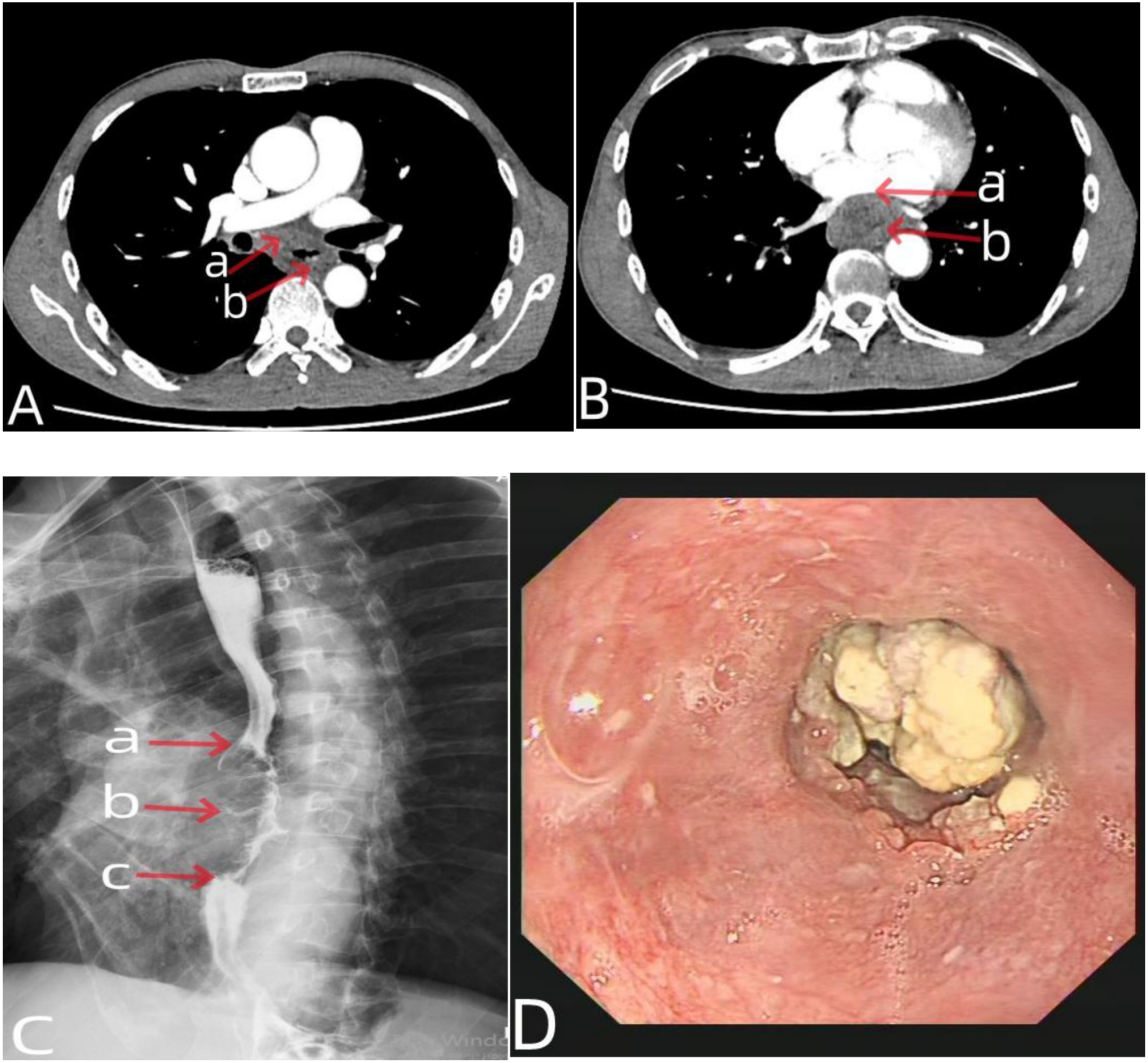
Chest CT, esophagography, and gastroscopy data of the patient before neoadjuvant chemotherapy. In (A), the arrow a represented enlarged subcarinal lymph nodes, the arrow b represented esophageal tumors. In (B), the arrow a represented the left atrium is compressed by tumor, the arrow b represented there was no clear boundary between the tumor and the thoracic aorta. In (C), the arrow a represented the upper edge of esophageal tumor, the arrow c represented the lower edge of esophageal tumor, and the arrow b represented the filling defect formed by esophageal tumors. In (D) showed a cauliflower like neoplasm in the esophageal lumen under endoscopy.
